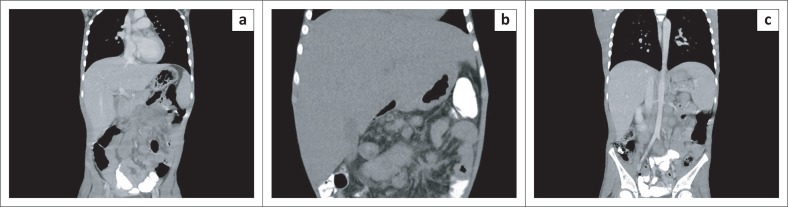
Radiology (computed tomography coronal images of the chest, abdomen and pelvis, a–c). Multiple conglomerate nodal masses are seen along the mesenteric, aorta, iliac and para-aortic nodal chains. There are also enlarged nodes in the porta hepatis. Several of the upper abdominal mesenteric nodes demonstrate low density, compatible with central necrosis. Diffuse hepatomegaly with no discrete lesion is seen.
